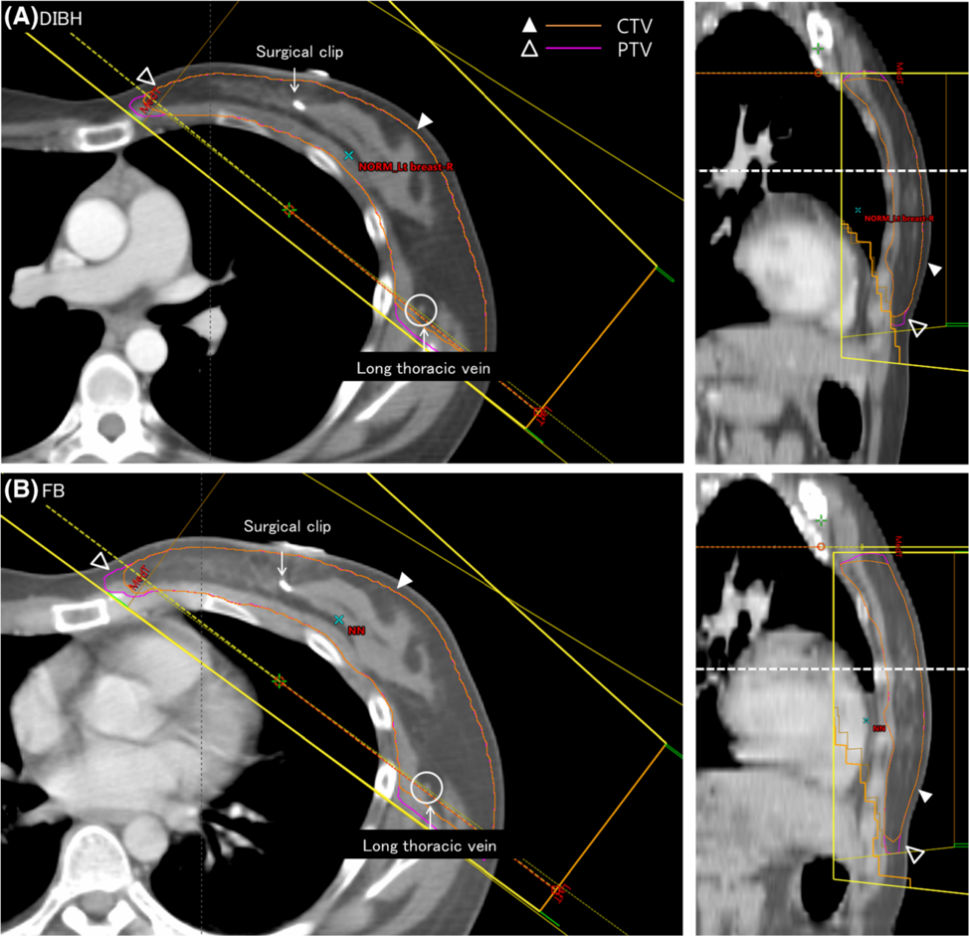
Axial computed tomography sections with deep inspiration breath holding (DIBH) (a) and free breathing (FB) (b) techniques. The anatomical matches of the CTV are confirmed by reference to the sternum and long thoracic vein. CTV, Clinical target volume; PTV, planning target volume
